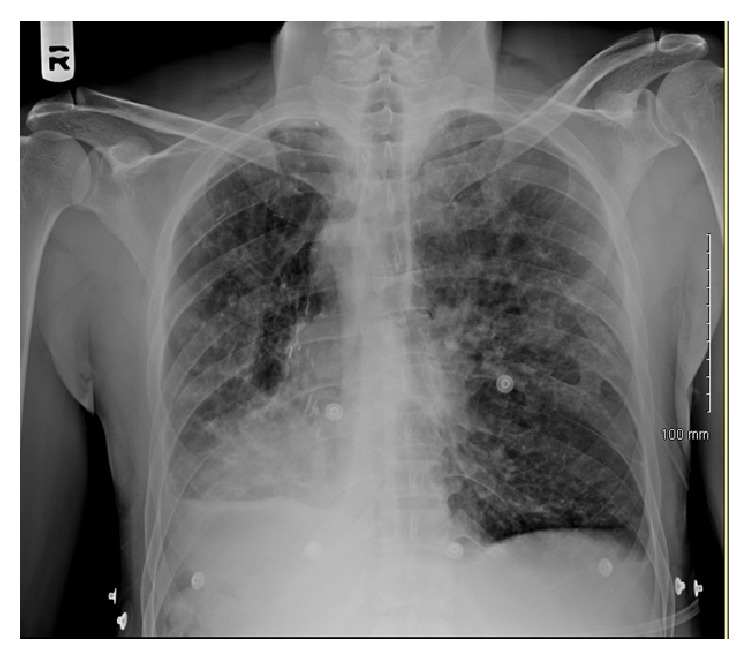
Chest radiograph showing dextrocardia and chronic interstitial markings from Kartagener's syndrome.
